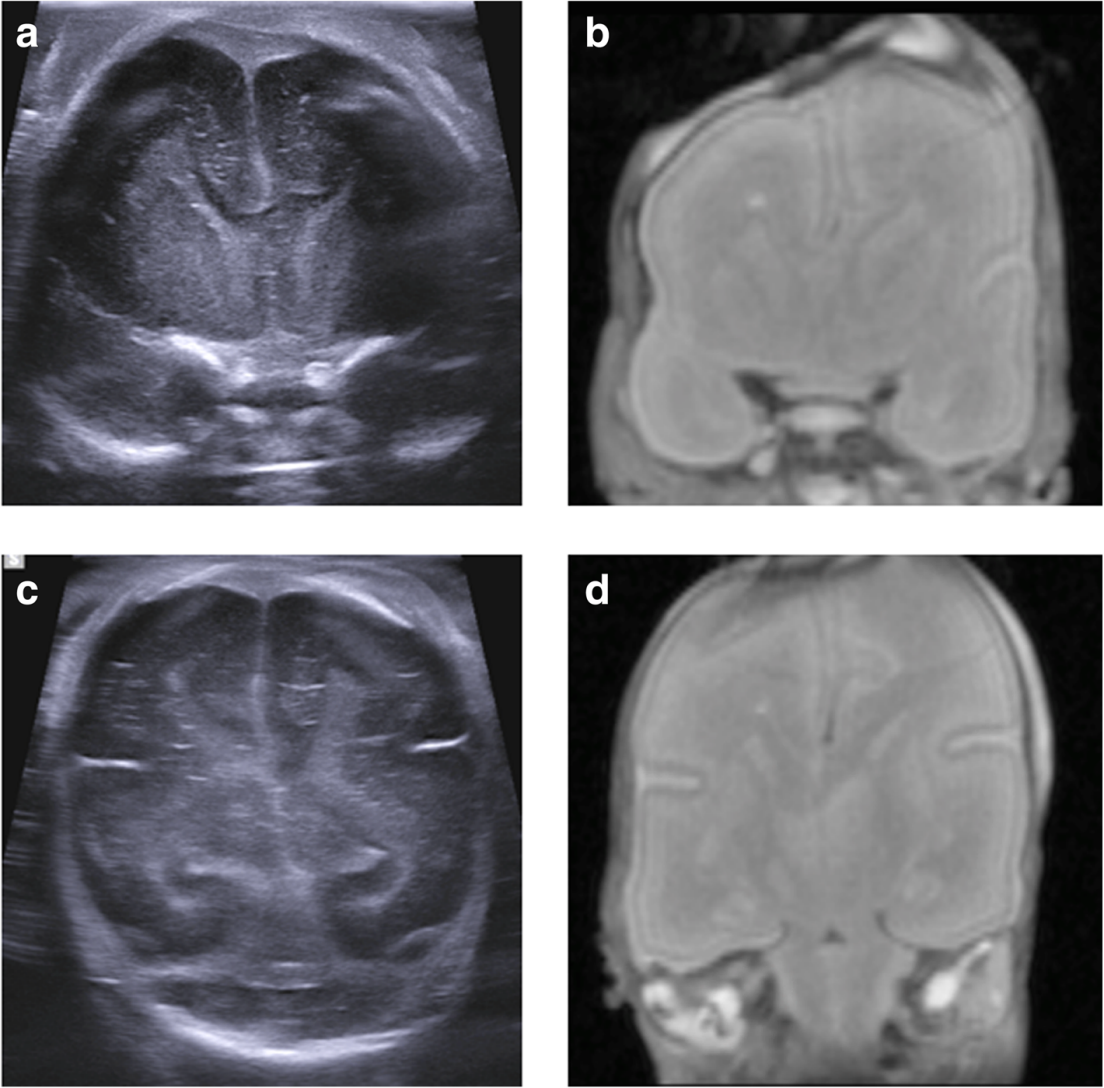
Paired coronal postmortem ultrasound images (obtained in the water bath) and T2-weighted postmortem MRI at 1.5 T in the same 20-week gestational-aged foetus. The images were obtained 4 days after death, demonstrating normal anatomy. Images through the frontal horns (a, b) and the posterior horns (c, d) of the lateral ventricles demonstrate how detailed anatomy of the brain, including sulcation and appearances of the ventricles can be adequately reviewed by ultrasound, and not necessarily require further cross-sectional imaging
